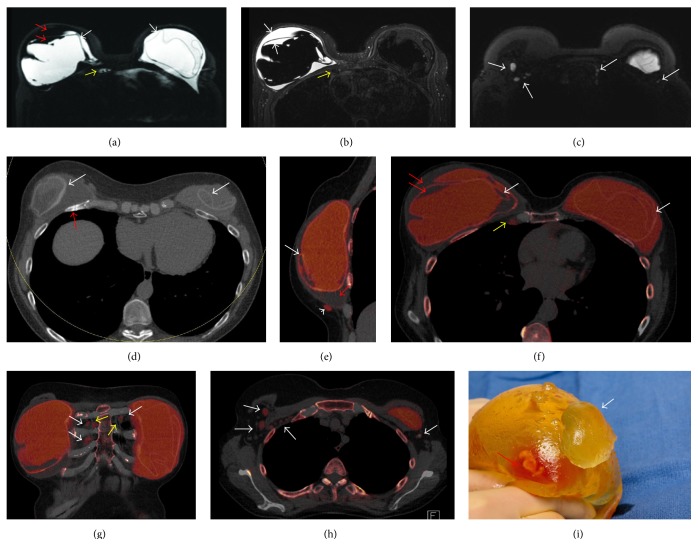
62-year-old woman presents with enlarging right breast following a fall one month earlier and a history of long standing bilateral subpectoral silicone implants following left modified radical mastectomy and simple right mastectomy in 1992. (a) Axial silicone sensitive MR sequence shows bilateral MR linguini sign of intracapsular rupture (white arrows) with low signal intensity material both within the capsule itself, along with high signal intensity silicone, and within the collapsed right envelope (red arrows). The high signal intensity tissue adjacent to the right internal mammary (IM) vessels (yellow arrow) was not appreciated as being likely due to silicone due to the inhomogeneity of the fat and water suppression about heart. (b) T2 weighted axial IDEAL MR sequence shows high T2 signal fluid of intracapsular seroma within the right envelope (white arrows). There is low signal intensity tissue adjacent to the right IM vessels which in retrospect would be in keeping with silicone within an internal mammary node (yellow arrow). (c) Axial silicone sensitive sequence from the high axilla demonstrates high signal intensity material which was not appreciated prospectively as right intranodal silicone in level I and II nodes and left IM nodes (arrows). (d) Axial mixed energy CT shows the CT linguini sign of collapsed implant envelopes bilaterally (white arrows). Healing fractured right anterior rib is clearly seen (red arrow). (e) Sagittal dual-energy noncontrast CT of the right breast with silicone colored as red shows intracapsular rupture with CT equivalent linguini sign of collapsed silicone envelope (white arrow). Water density material (red arrows) is noted surrounding the collapsed right envelope as seen on fluid sensitive MRI sequence (c). Extracapsular silicone is noted inferiorly (arrowhead). (f) Axial dual energy noncontrast CT of the right breast with silicone colored as red shows bilateral intracapsular rupture with CT equivalent linguini sign of collapsed silicone envelopes (white arrows). Water density material (red arrows) is noted surrounding and within the collapsed right envelope as seen on fluid sensitive MRI sequence (c). Silicone is also easily seen within enlarged right IM lymph node (yellow arrows). (g) Coronal DECT with color coding shows silicone within the right and left internal mammary nodes (white arrows). The internal mammary vessels can be seen (yellow arrows). (h) Axial DECT with silicone colored as red show silicone within right level I and II and left level I nodes (arrows). (i) Photograph of the explanted right implant with extruded silicone from the envelope (white arrow). Serosanguineous fluid noted within the envelope as seen on MRI and CT (red arrow).
